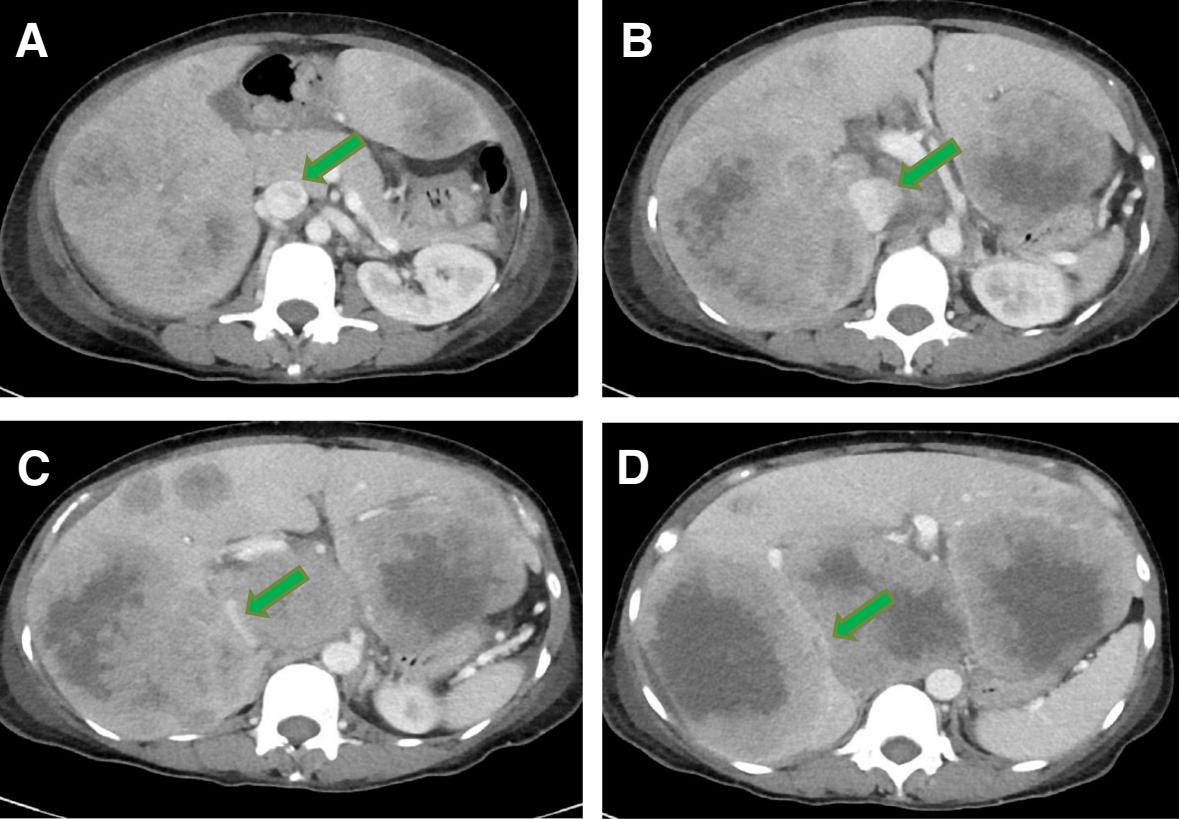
Compression of the inferior vena cava by foci of hepatic metastases. Computed tomographic scan of the abdomen and pelvis with intravenous contrast shows that the inferior vena cava (*green arrows*) becomes progressively more compressed by the tumor superiorly to inferiorly. a Patent inferior vena cava at the level of the pancreas. d Near-complete compression of the inferior vena cava at a level of the superior portion of the kidneys. a-d Superiorly to inferiorly
